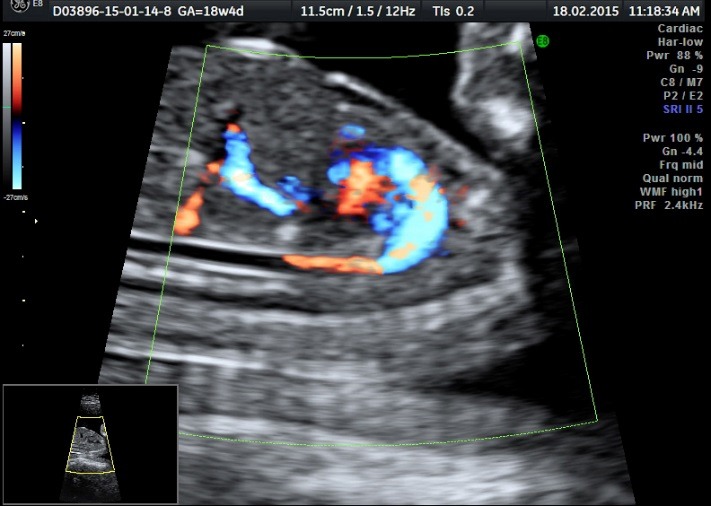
Normal fetus (pumping twin) with a normal fetal blood flow – Doppler examination of the Aortic arch
